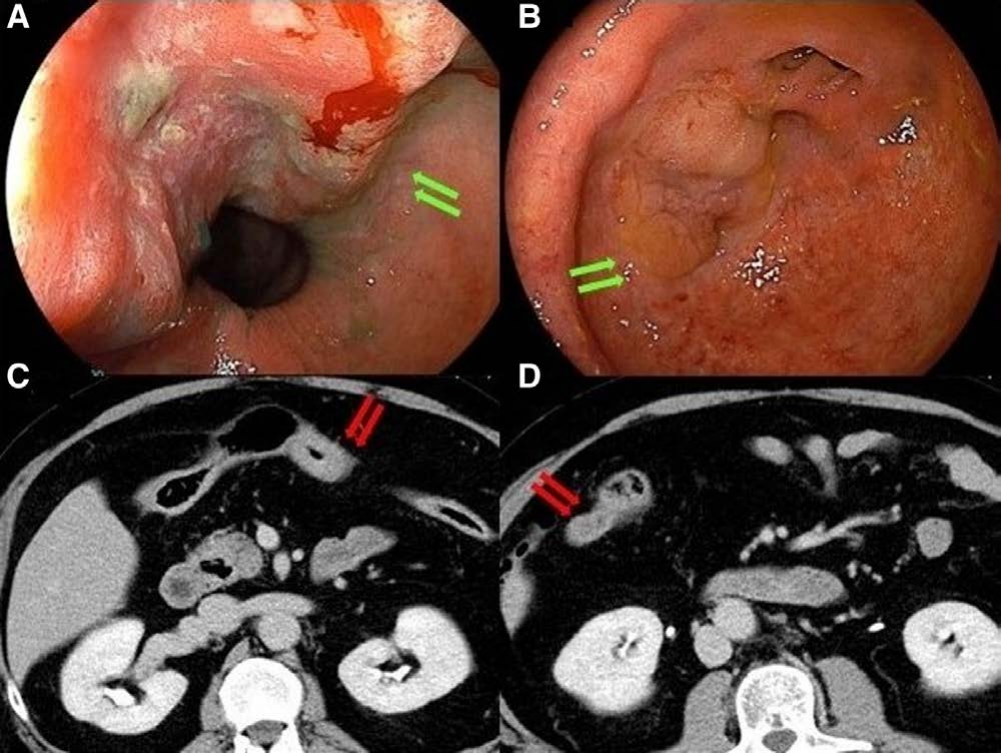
Preoperative examination findings. (A) Colonoscopy findings reveal a type 3 advanced tumor located in the mid-transverse colon (arrow). (B) Colonoscopy findings reveal a LST-like lesion located around the appendiceal orifice in the cecum (arrow). (C) Abdominal contrast-enhanced computed tomography reveals the presence of a tumor within the transverse colon (arrow). (D) Abdominal contrast-enhanced computed tomography findings also reveal an enlarged appendix (arrow). LST = laterally spreading tumor.
